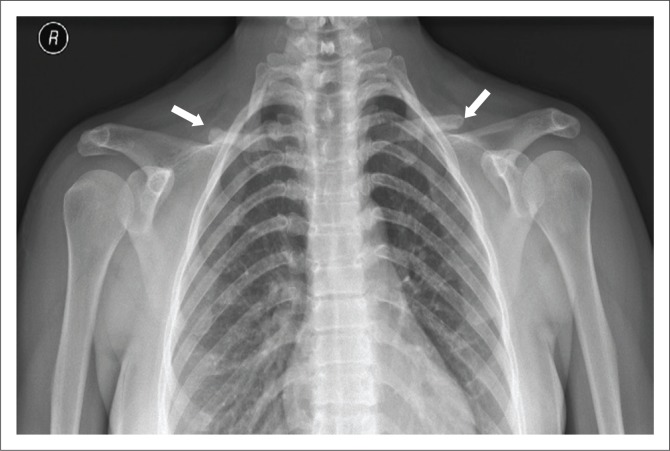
Postero-anterior radiograph of the upper chest shows aplasia of lateral two-thirds of both clavicles (white arrows) and a bell-shaped thorax.
